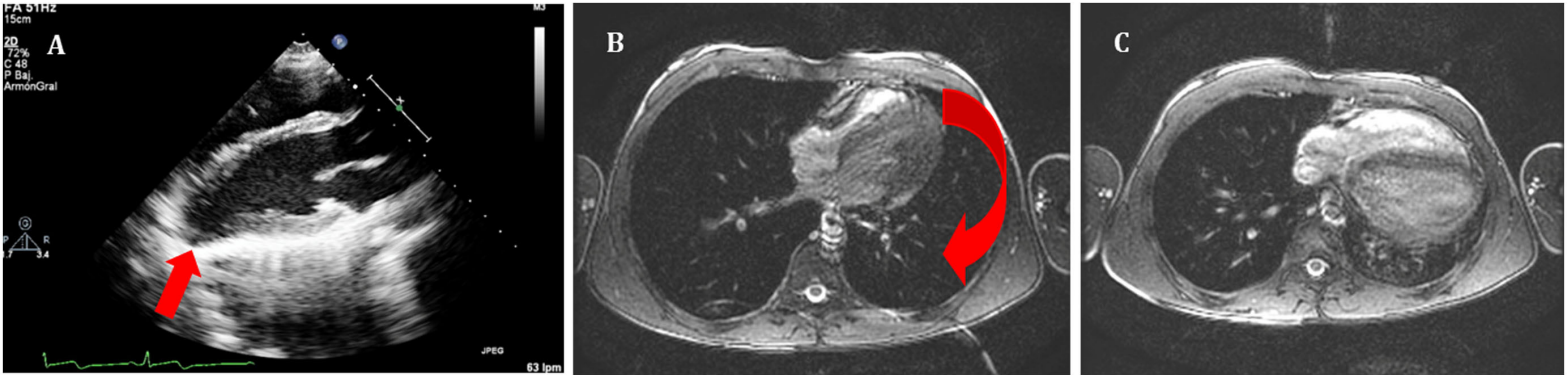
(A) Echocardiography in parasternal long axis. Arrow shows the posterior displacement of the apex and the lack of the typical round left ventricular apex. Cardiovascular magnetic resonance showing the clockwise rotation. Compared with the prone position (B), leftward posterior rotation of the heart is seen in when patient is in supine position (C).
